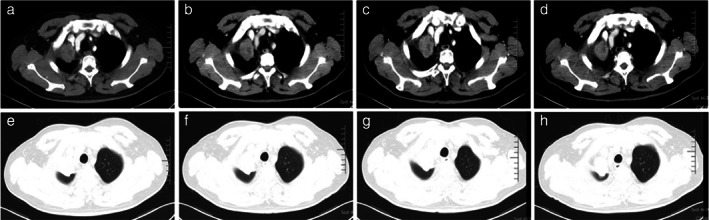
After radiotherapy and chemotherapy, the overall effect of the treatment was evaluated as stable disease by comparing CT images acquired on July 10 (a and e), August 7 (b and f), September 29 (c and g), and November 16, 2020 (d and h)
